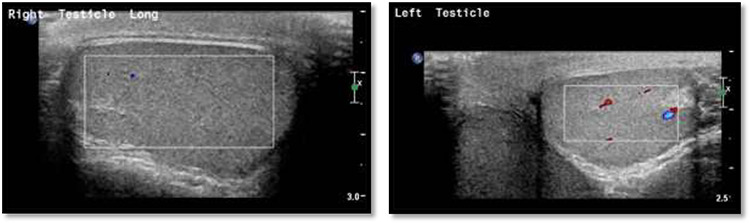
Scrotal Doppler ultrasound shows the size, echogenicity and vascularity is maintained for both testicle with slightly reduced in the right testicle that could be suggestive of right torsion/detorsion.
